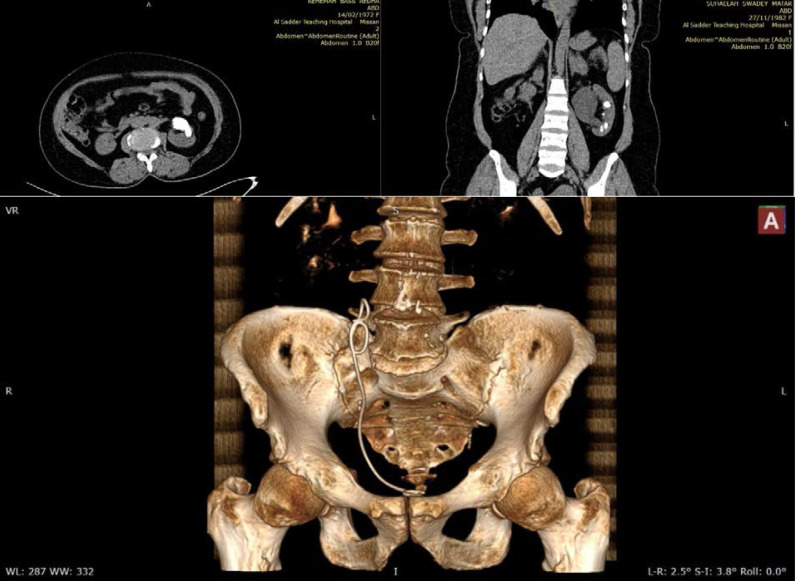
CT scan images of renal stone
